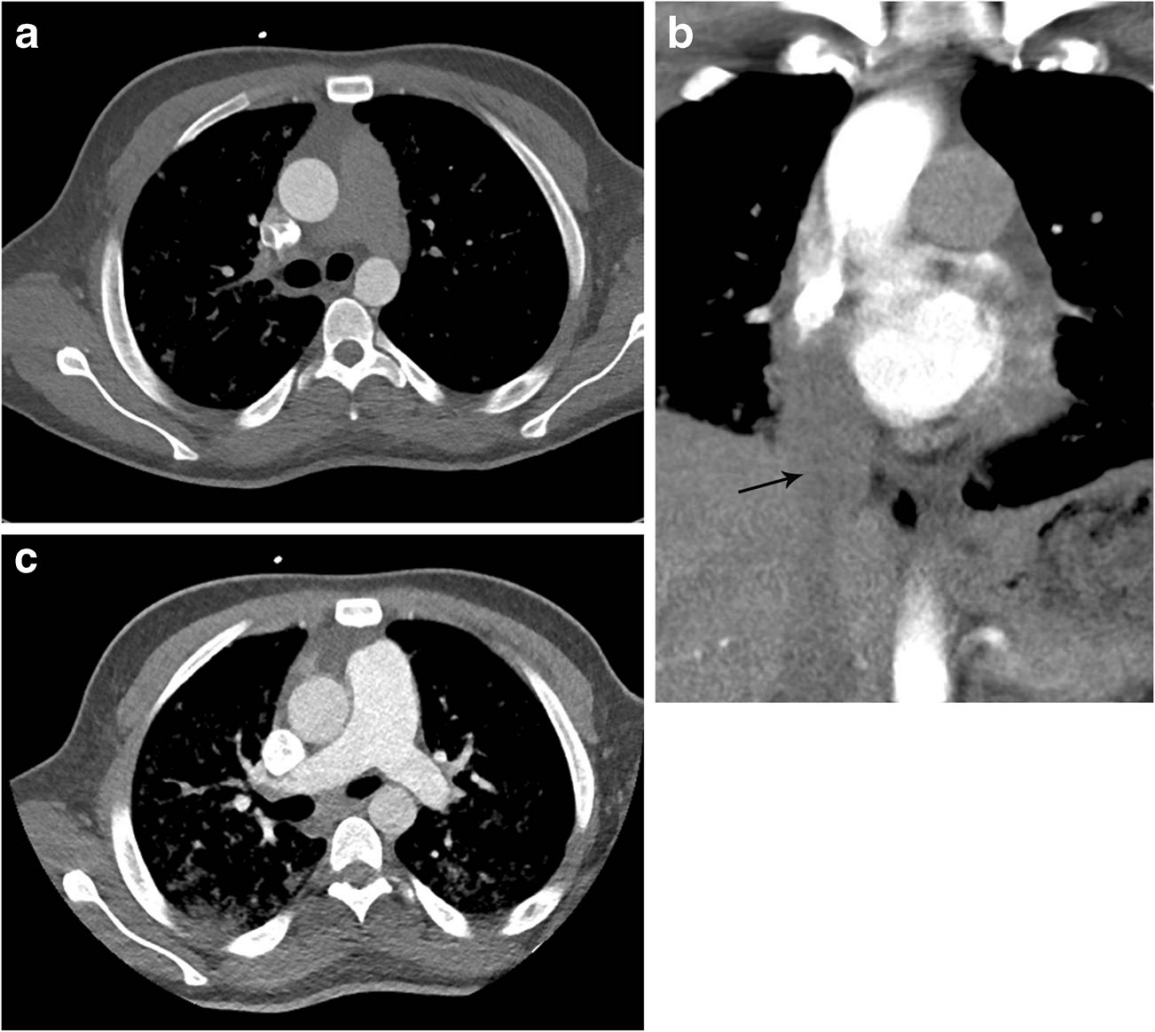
Transient interruption of contrast bolus results in suboptimal opacification of the pulmonary artery on initial contrast bolus, with subsequent diagnostic scan for pulmonary embolus after repeat injection using high pitch FLASH CTA. The initial CECT (a) shows poor opacification of the pulmonary artery despite high density contrast material within the aorta and SVC because deep inspiration resulted in increased venous return from the IVC and dilution of the contrast bolus in the right heart (b) at the time of scanning. Repeat injection using high pitch FLASH technique with free breathing (c) shows excellent opacification of the pulmonary artery
